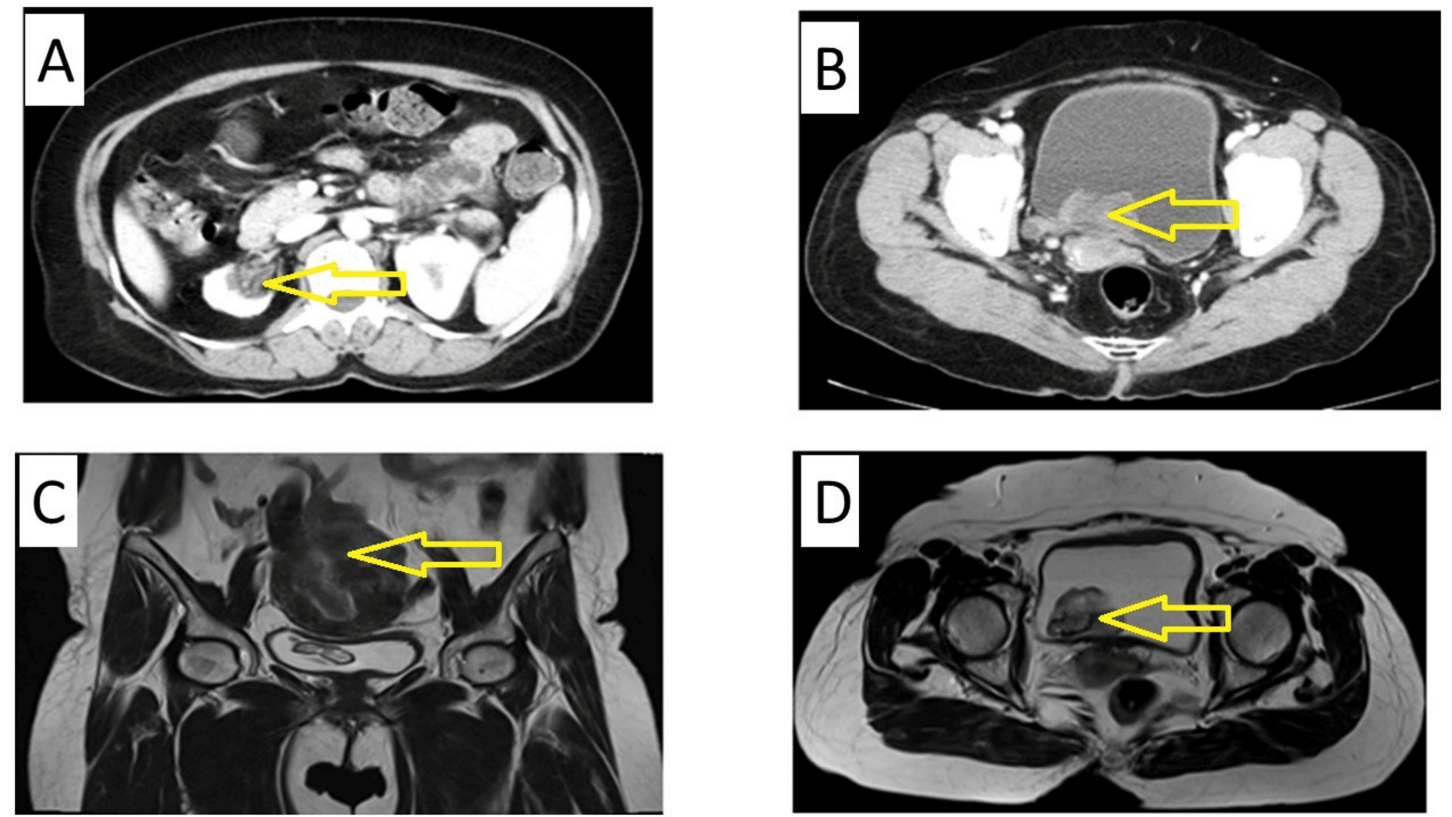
A CT image showing a tumor arising from the right renal pelvis running through the entire right ureter extruding into the bladder (A) from the right ureteric orifice (B) and MRI images showing the tumor extruding into the bladder lumen (C) and (D). CT: computed tomography; MRI: magnetic resonance imaging
